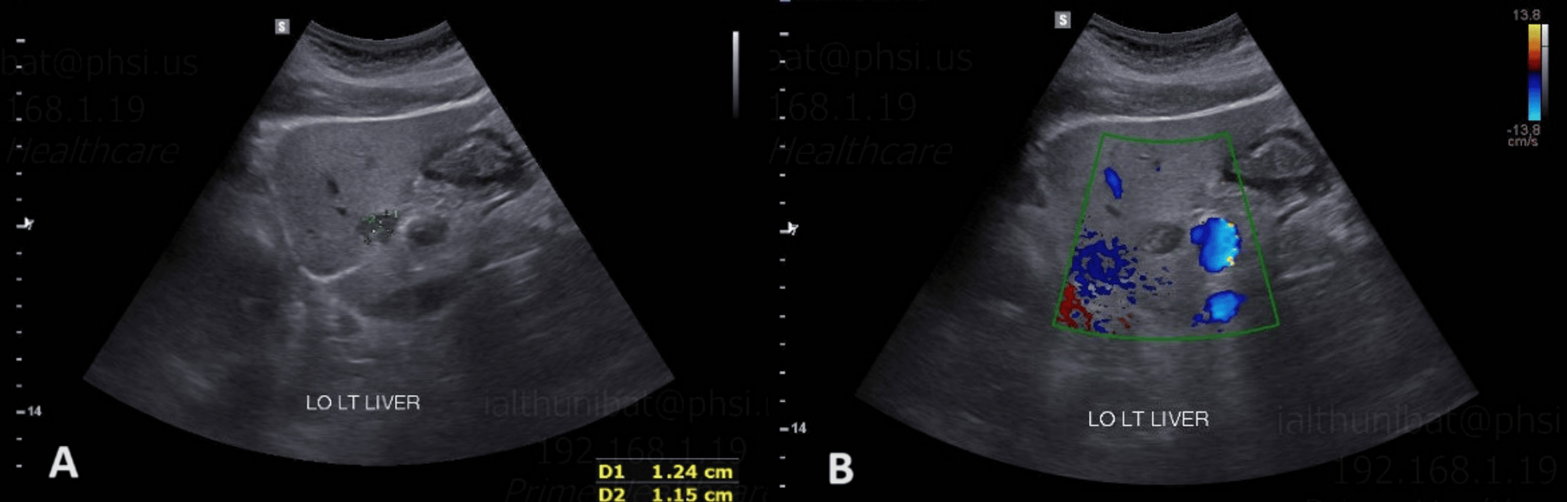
The well-circumscribed lesion in the left hepatic lobe (A), which showed hypovascularity on Doppler imaging (B).
